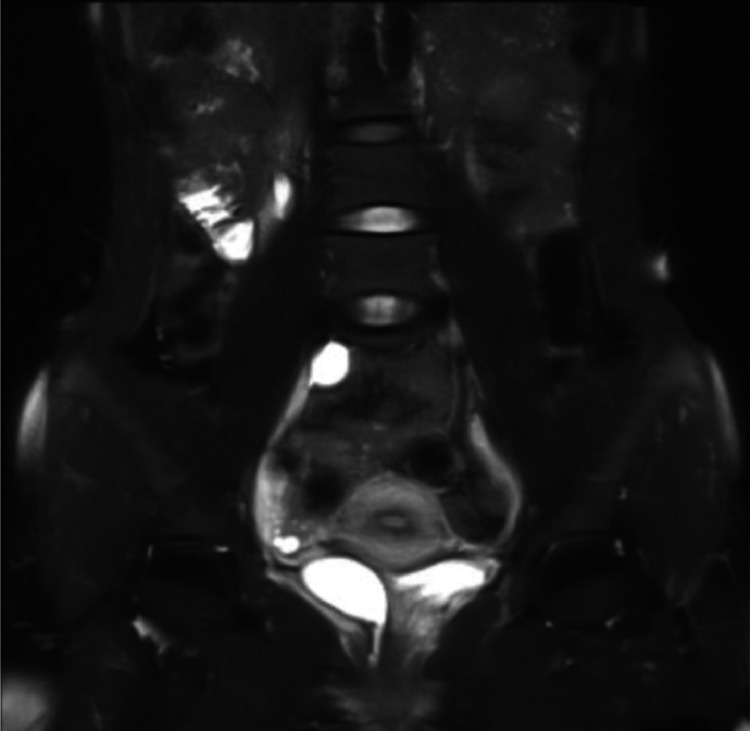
MRI T2 sequence coronal.
